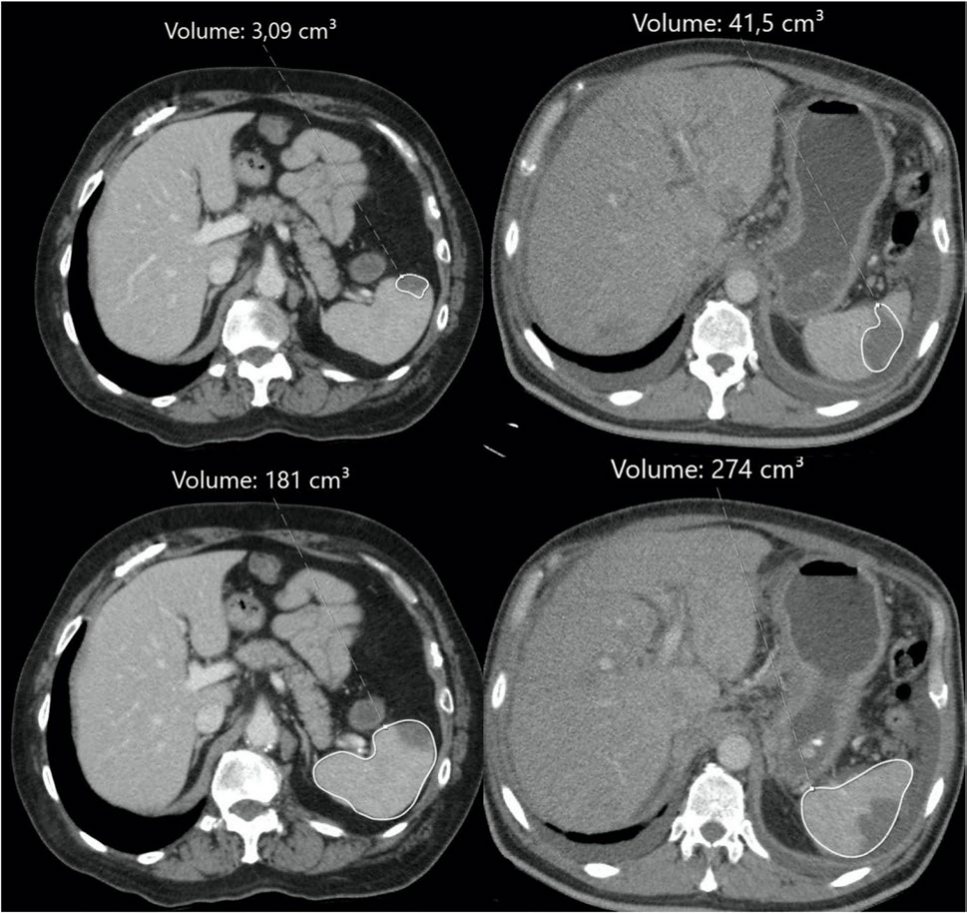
Axial contrast-enhanced abdominal CT images demonstrating splenic infarction with volumetric analysis
